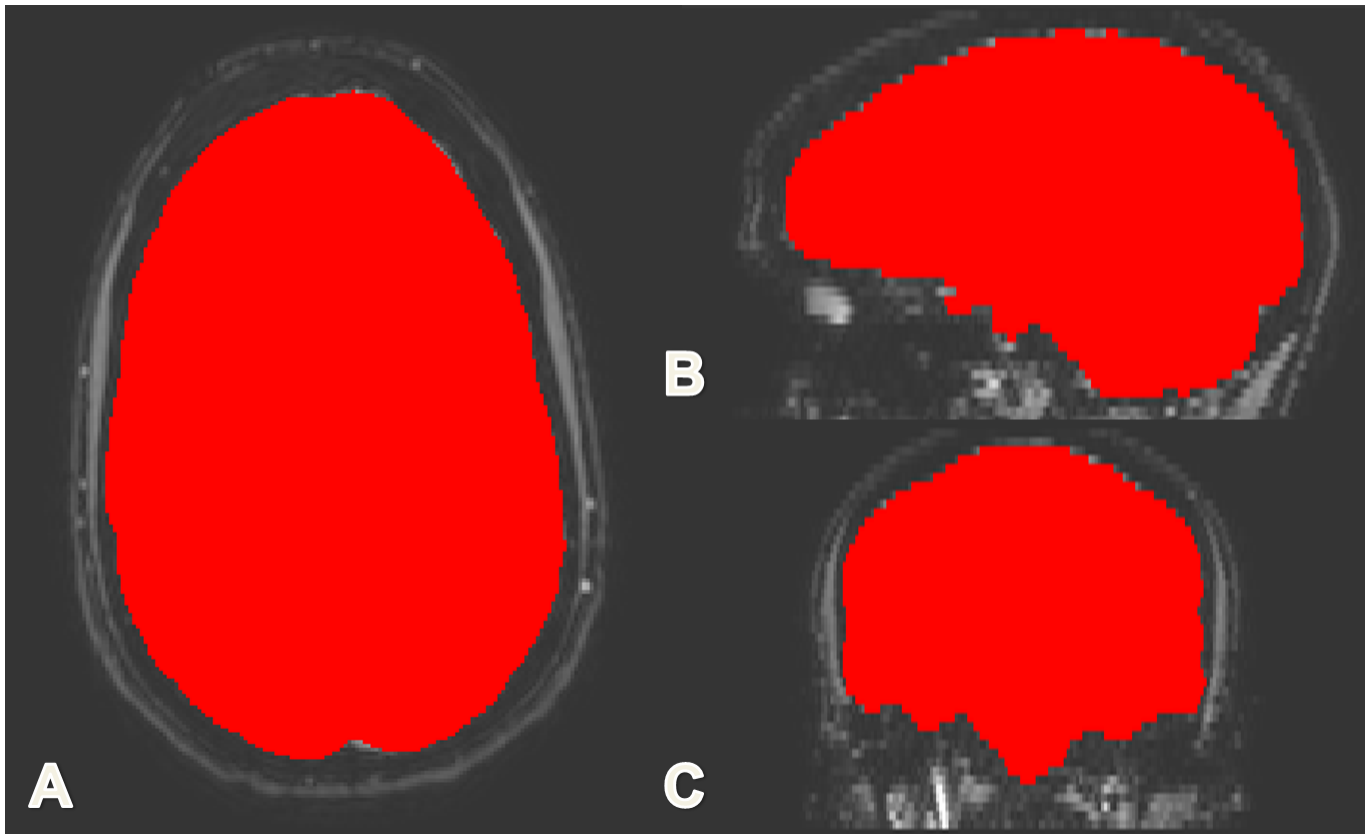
The CSF MRI sequence. After presaturation and T_s_ the signal strength depends on T_1_ decay to ensure an equal magnetization at the start of each MLEV-T_2_ preparation. The MLEV-T_2_ preparation with a varying amount of refocusing pulses allows the mapping of T_2_ decay. Variation of time T_s_ in combination with a fixed number of refocusing pulses allow the mapping of T_1_ decay. A short T_s_ is used to determine signal decay. Multi-slice echo planar imaging is used for the readout at each effective echo time.
